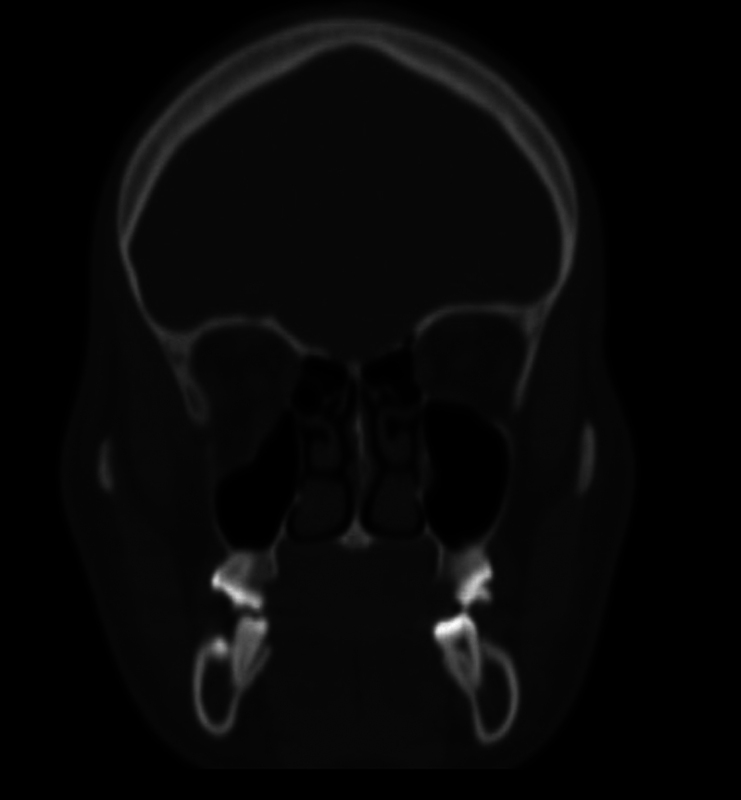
Computed tomographic (CT) scan of coronal posterior view.
